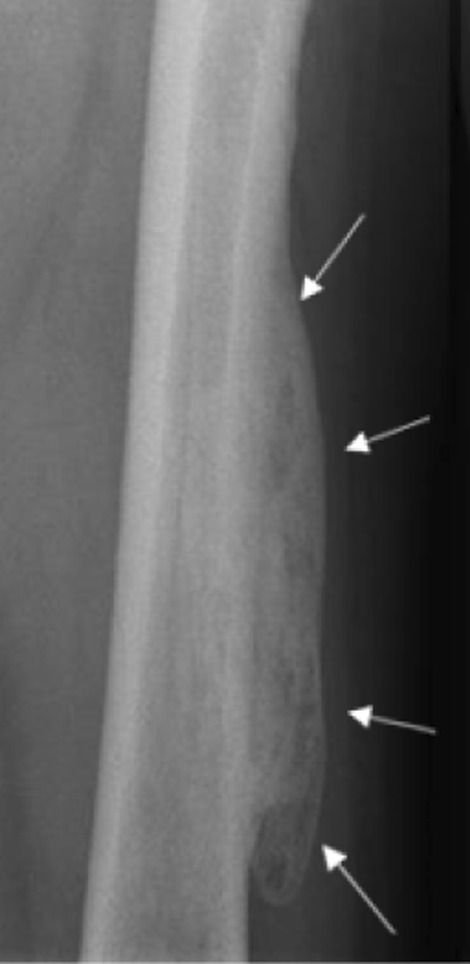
Plain radiograph at the mature stage shows global ossification of the mass (arrows) with complete incorporation into the femoral cortical bone
